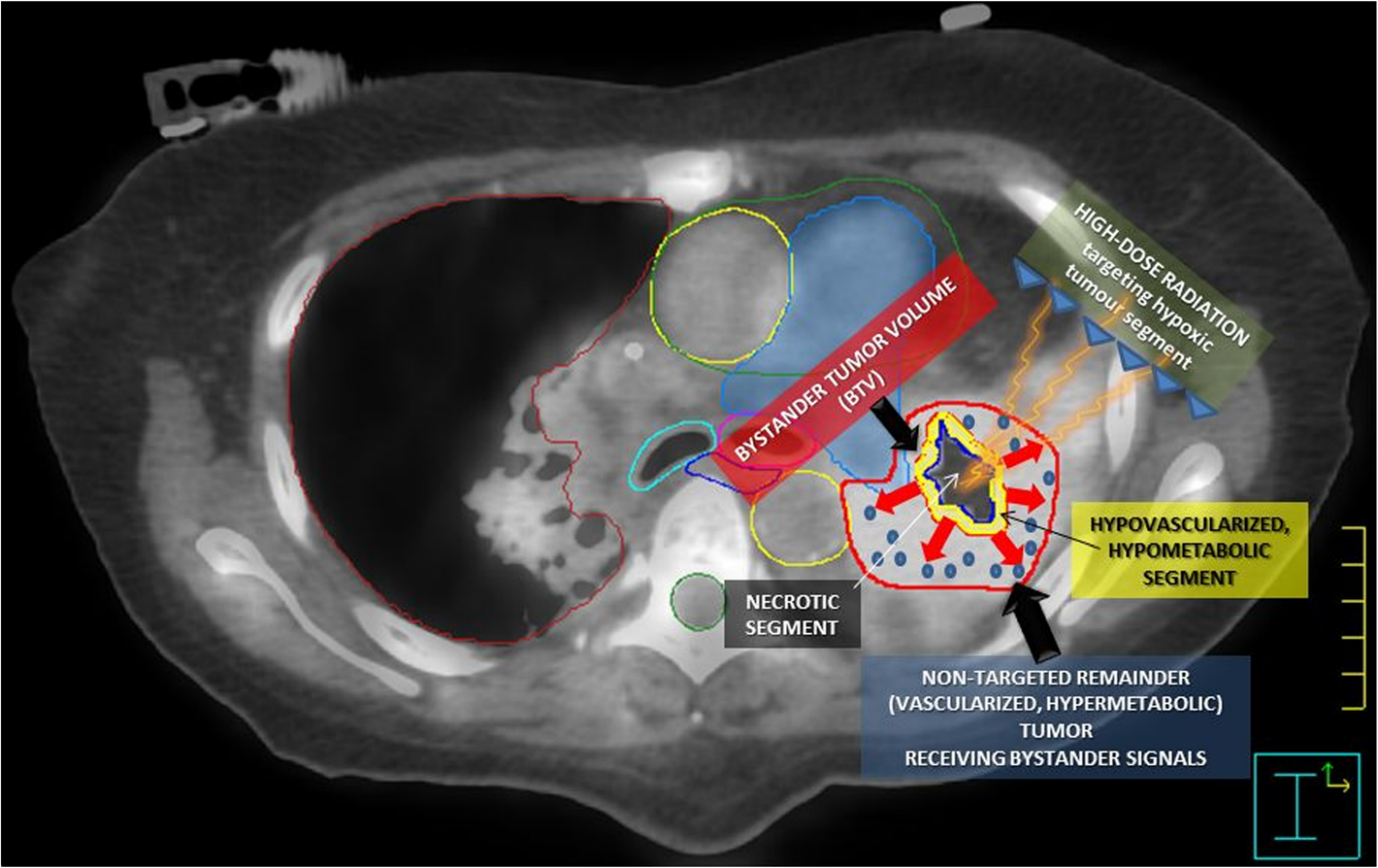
DEFINITION OF THE BYSTANDER TUMOR VOLUME (BTV): The figure summarizes the radiobiology of the bystander effect-induction by SBRT-PATHY. An 18F-FDG PET combined with a contrast-enhanced CT was used for the definition of BTV (smaller yellow contour), which corresponds to the junctional region between the central necrotic segment (black region) and the contrast-enhanced, hypermetabolic peripheral tumor (red contour, not targeted for irradiation). The red arrows represent “anti-angiogenic bystander signal” (blue pellets) released by the irradiated hypoxic tumor, inducing the regression of the non-targeted tumor
